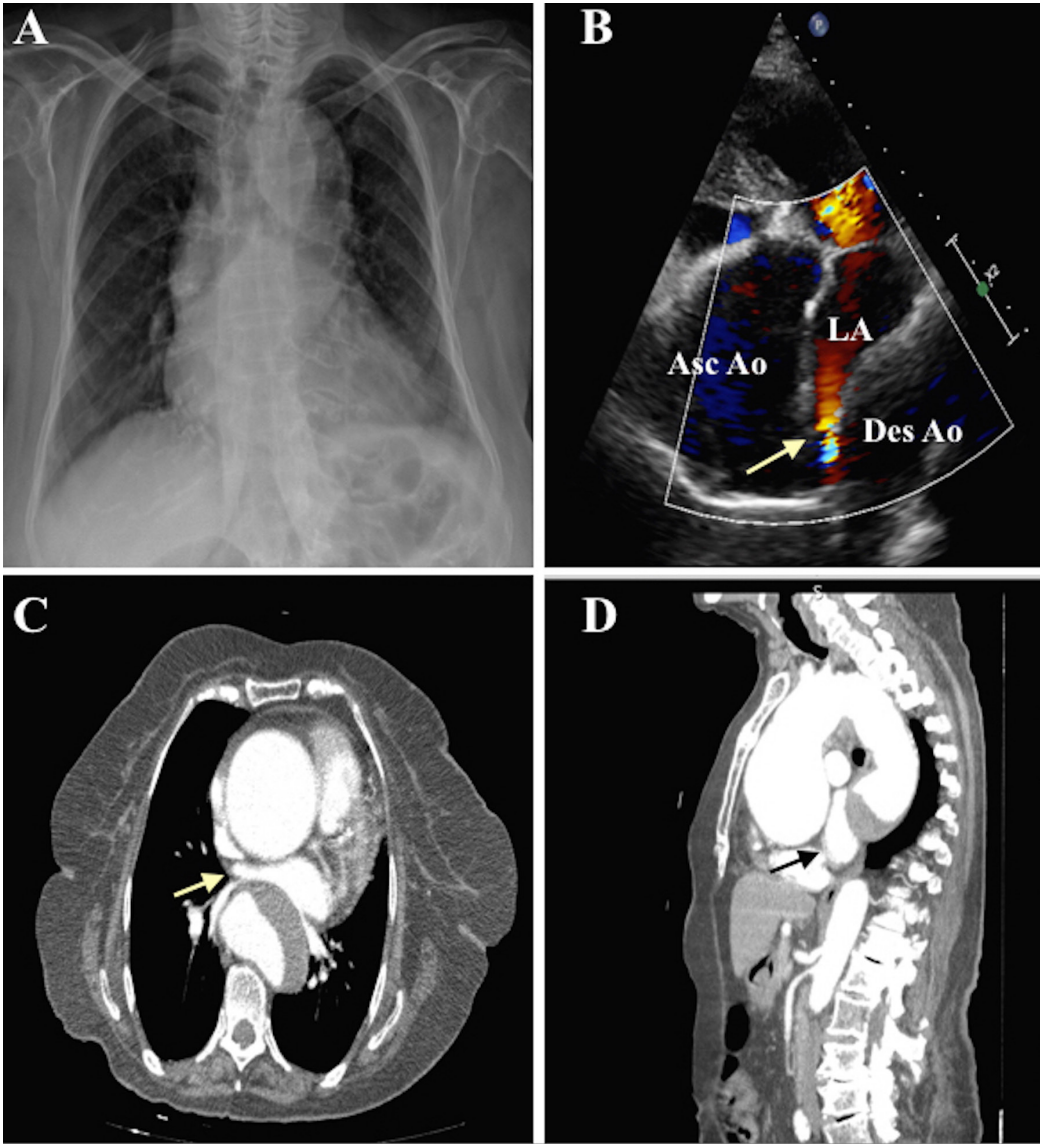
A) Chest X-ray demonstrated a widened mediastinum. B) Transthoracic echocardiography in modified apical 5-chamber view showed that left atrium was compressed between ascending and descending aortas, and color Doppler turbulence was seen in the compressed area (arrow). C) A contrast-enhanced chest computed tomography scan in axial plane showed that left atrium and pulmonary veins were compressed between ascending and descending aortic aneurysms. D) A contrast-enhanced chest computed tomography scan in coronal plane showed that left atrium and pulmonary veins were compressed between ascending and descending aortic aneurysms.
